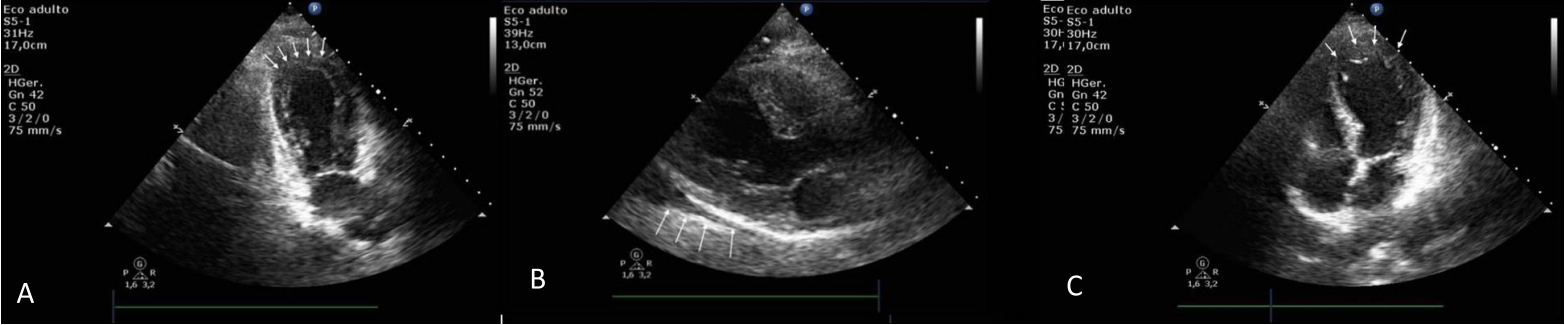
Apical 4 chambers view showing akinesia of the mid-apical segments of the inferior septal and anterolateral walls, determining apical ballooning (arrows). LA: Left Atrial. LV: Left Ventricle. RA: Right Atrial. RV: Right Ventricle
